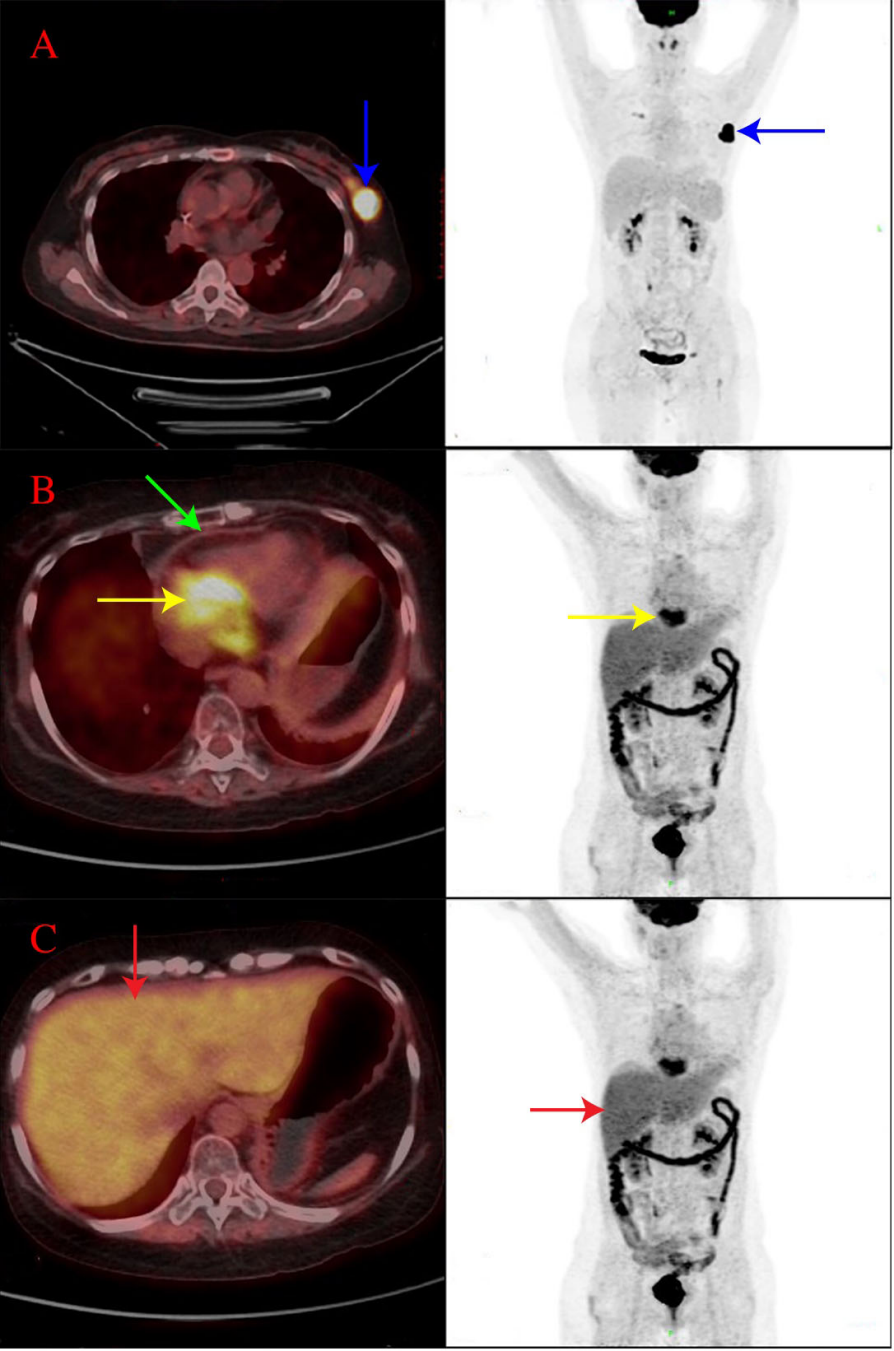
PET-CT findings of the patient. (A) In September 2020, there was an irregular soft tissue mass in the upper outer quadrant of the breast (4.1 × 2.0 cm), with an SUV_max_ of 23.0, as shown by the blue arrow. (B) In November 2021, there were a cardiac mass (6.0 × 4.4 cm), with an SUV_max_ of 10.5, as shown by the yellow arrow and a diffusely thickened pericardium, with an SUV_max_ of 2.0, as shown by the green arrow. (C) In November 2021, there was an abnormal uptake in the liver, with an SUV_max_ of 5.0 and a Deauville score of 5 points, as shown by the red arrow.
